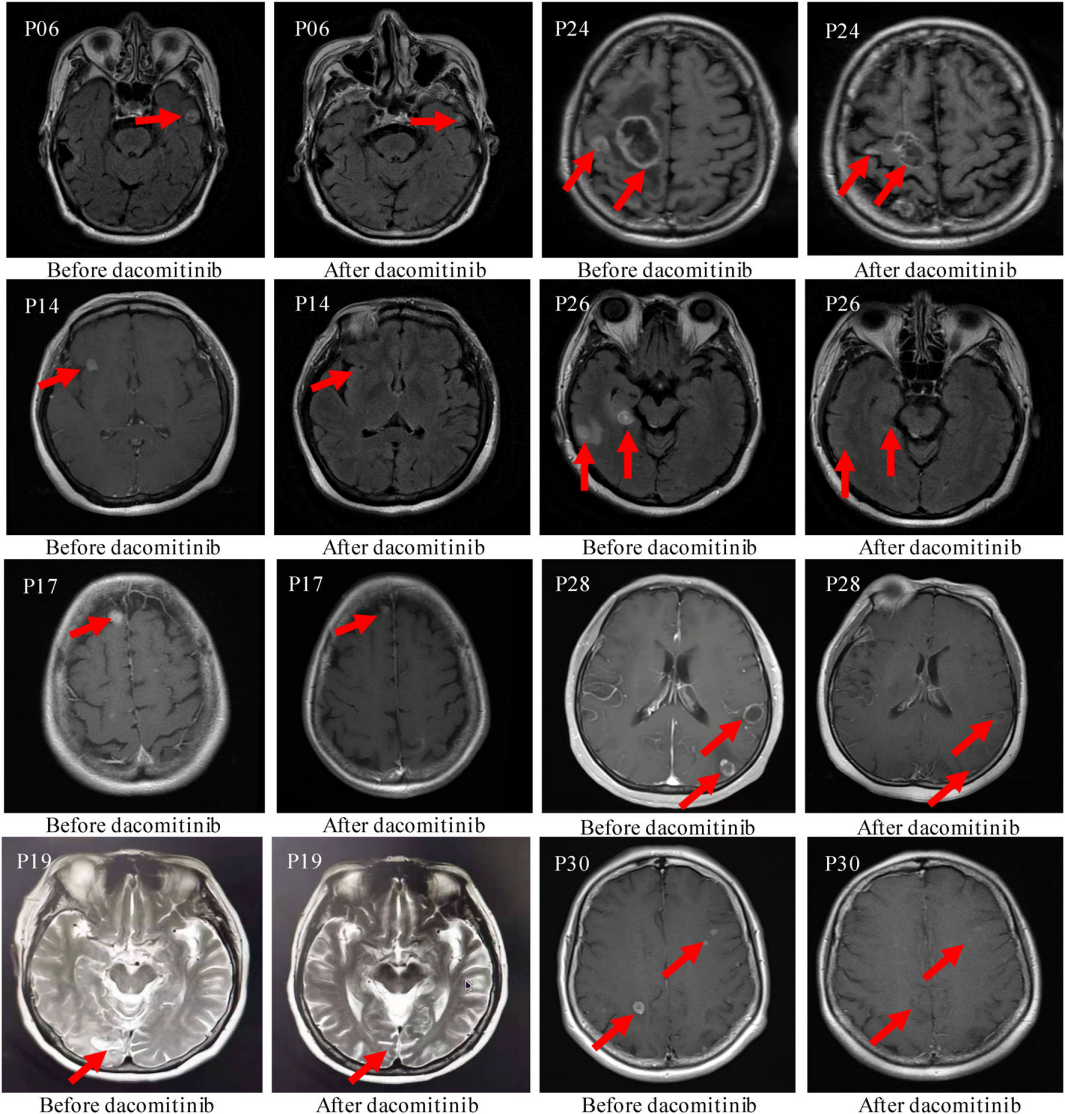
Brain MRI indicating the intracranial response to dacomitinib in patients with measurable lesions
